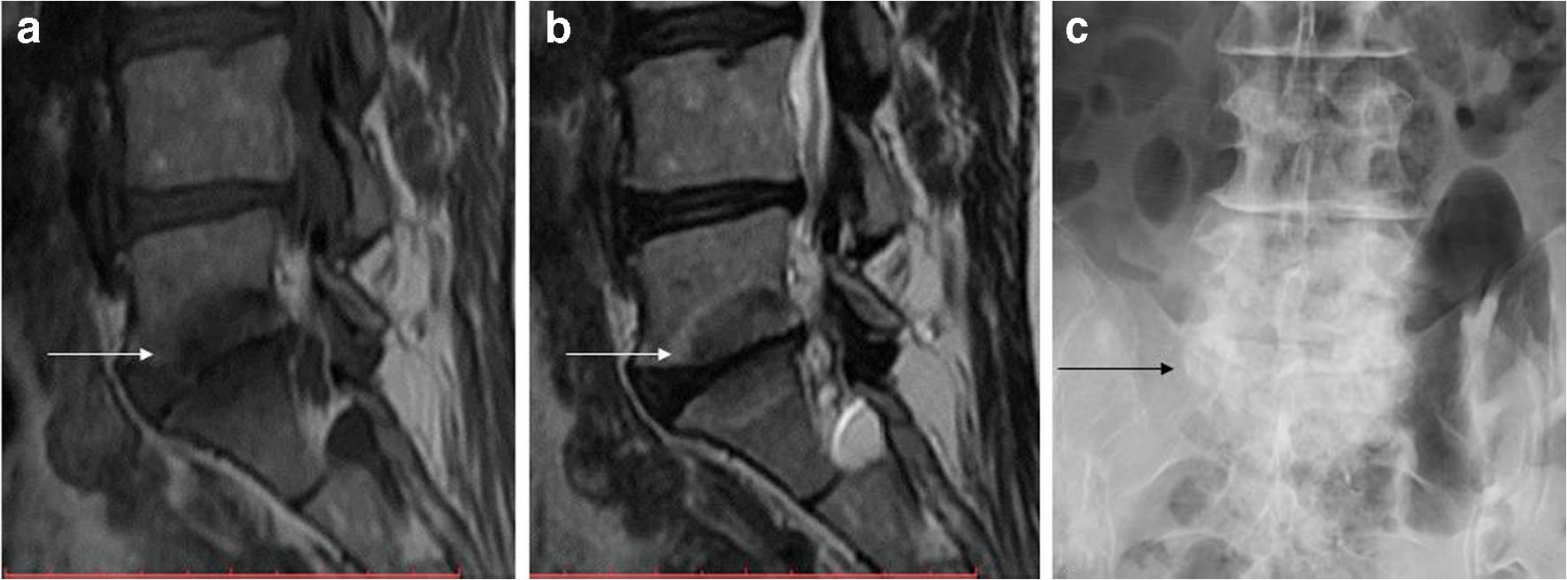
a, b, c Degenerative. Modic changes type III in a 53-year-old woman. The hypointense SI areas (*arrows*) both on T1w (A) and T2w (B) imaging reflect a subchondral bone sclerosis. The lumbosacral spine x-ray of the same patient (C) allows to better observe the bone sclerosis (*arrow*) of the caudal lumbar spine (courtesy of Giacomo Aringhieri, MD, University of Pisa School of Medicine, Italy)
